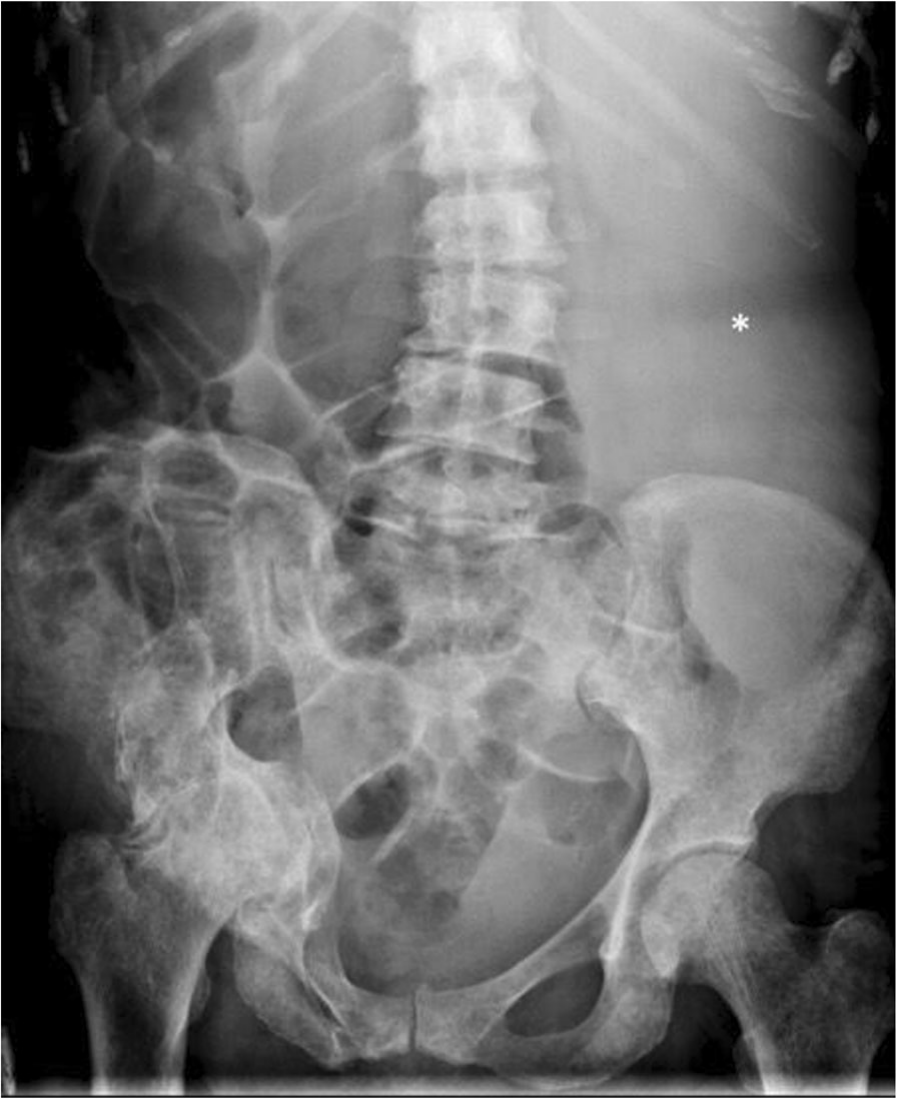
Plain abdominal radiograph demonstrating diffuse increased osteosclerosis and a large left upper quadrant shadow consistent with massive splenomegaly (asterisk) in a patient with known primary myelofibrosis. There is marked displacement of the large and small bowel to the right by the spleen
